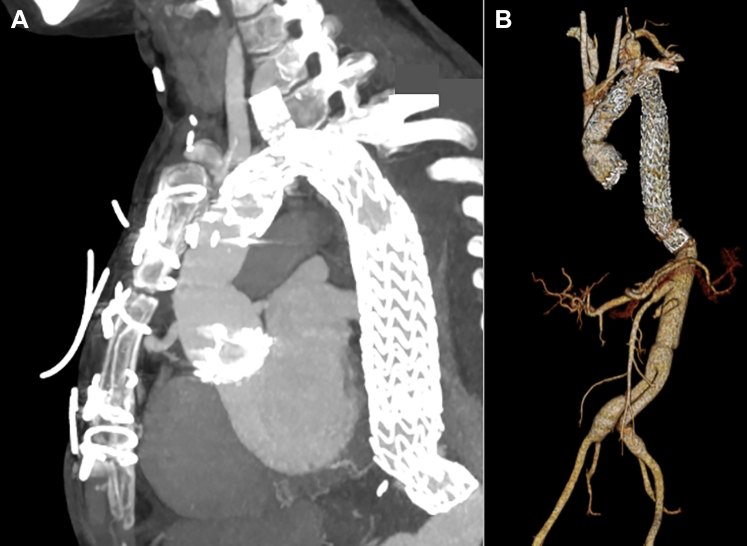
Postoperative Surveillance CTA After TEVAR Postoperative CTA demonstrated (A) thoracic branched endograft without endoleak and (B) complete aortic replacement from the aortic root to the bilateral iliac arteries. CTA = computed tomography angiography; TEVAR = thoracic endovascular aortic repair.
